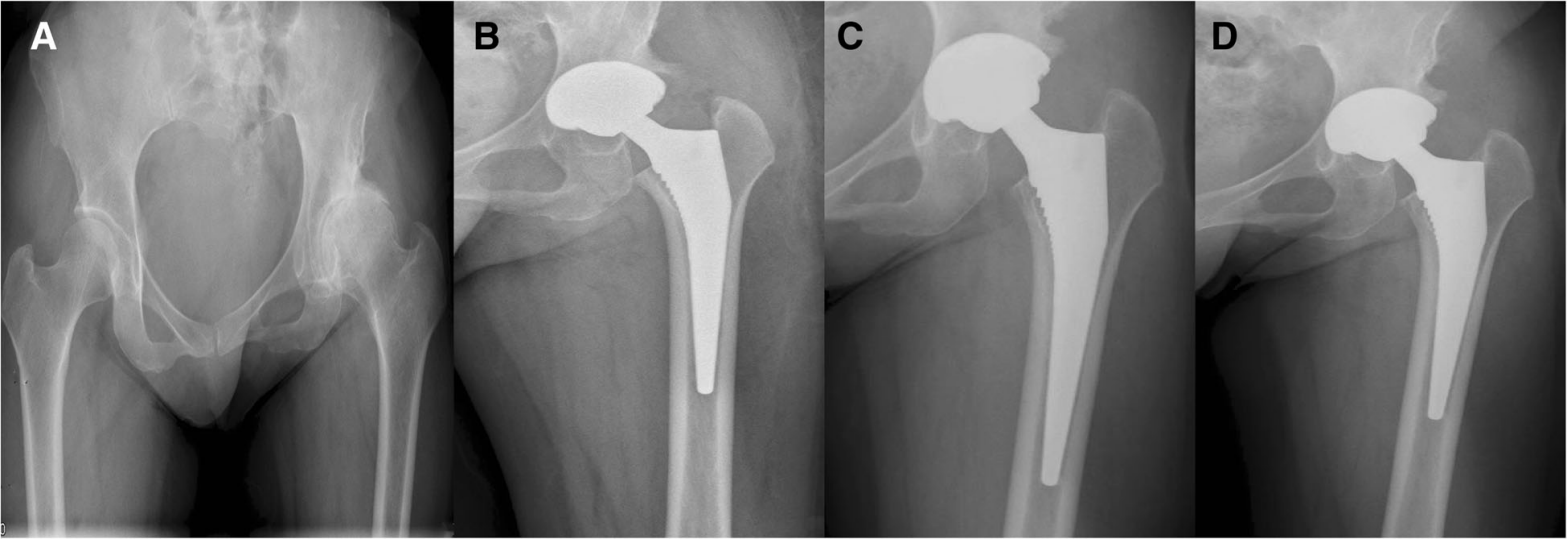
Radiographs of a 53-year-old woman with unilateral osteoarthritis secondary to hip pyogenic infection. a Preoperative anteroposterior view. b Postoperative imaging. Total hip arthroplasty is used to reconstruct the hip at the anatomical central level. c At 4-year follow-up, no radiolucent lines around the femoral stem were found. d At 7-year follow-up, no subsidence or loosening was found, and the femoral and acetabular components were considered to be stable
